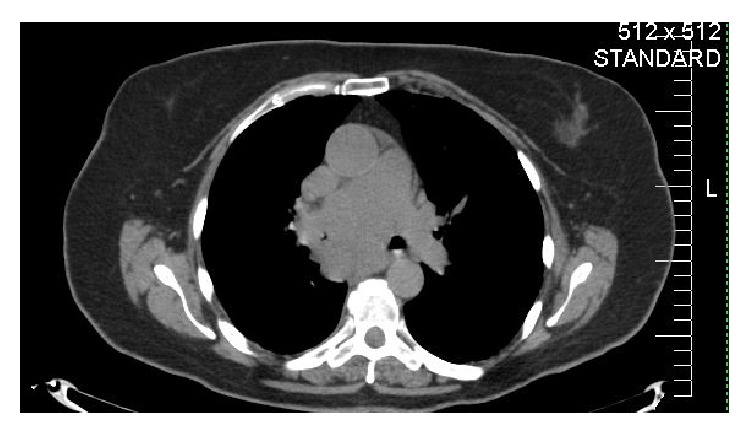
CT of right lung mass occluding the bronchus intermedius and a subcarinal mass which invades the left main bronchus.
